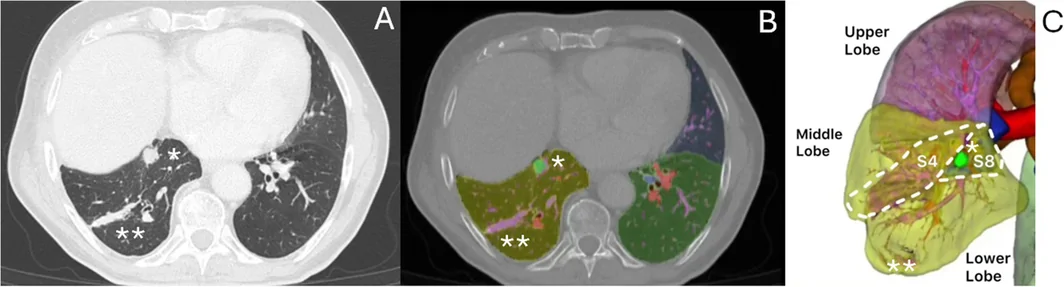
Chest computed tomography scan (A) and 3D segmentation (B) showed tumor (*) and previous resections (). 3D model (C) defined that prompt position of the tumor (*) and previous resection ().
